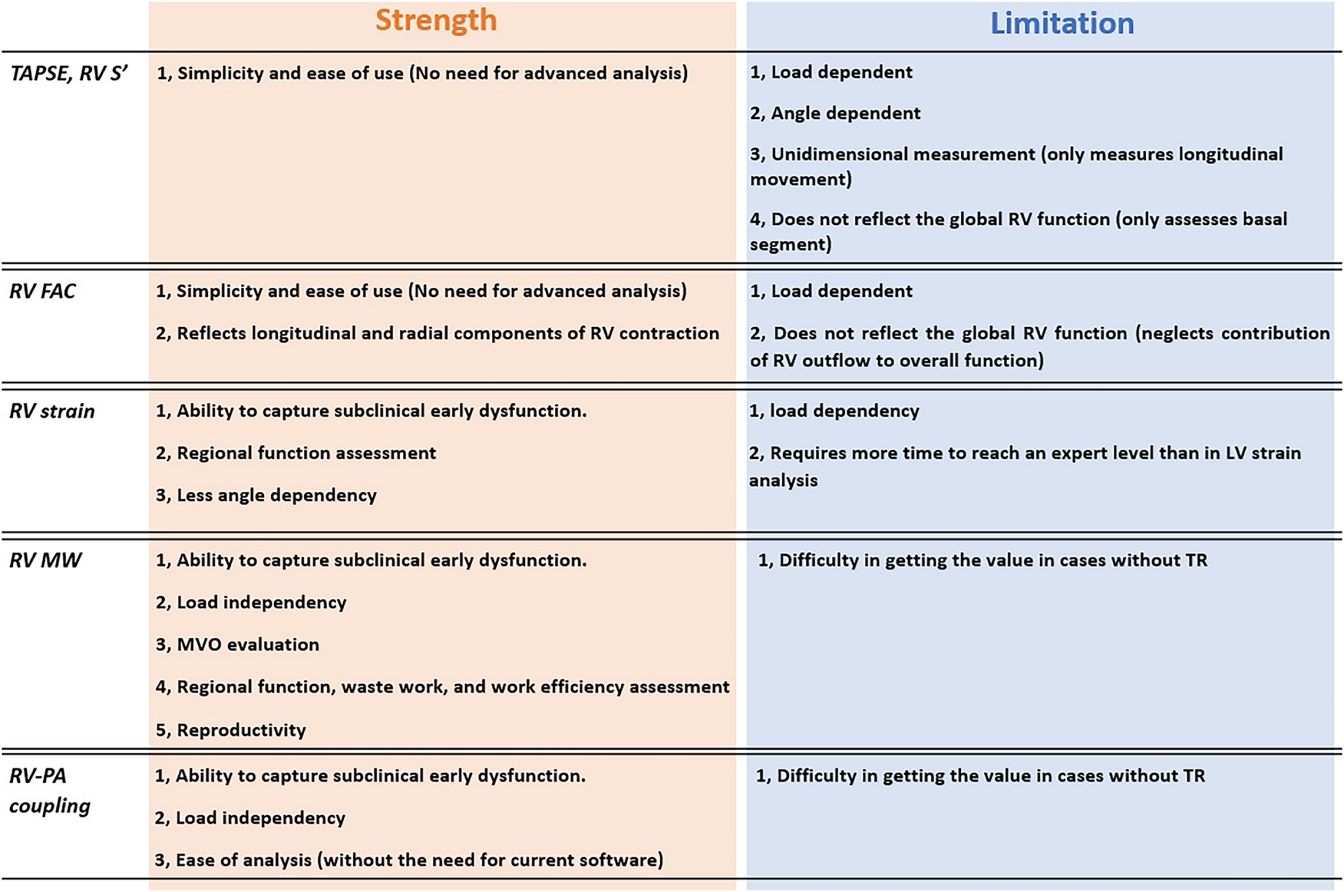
Strengths and limitations of novel and conventional RV echocardiographic parameters. RV, right ventricular; PA, pulmonary artery; RVD, right ventricular dysfunction; MVO, myocardial oxygen consumption; TR, tricuspid regurgitation.
